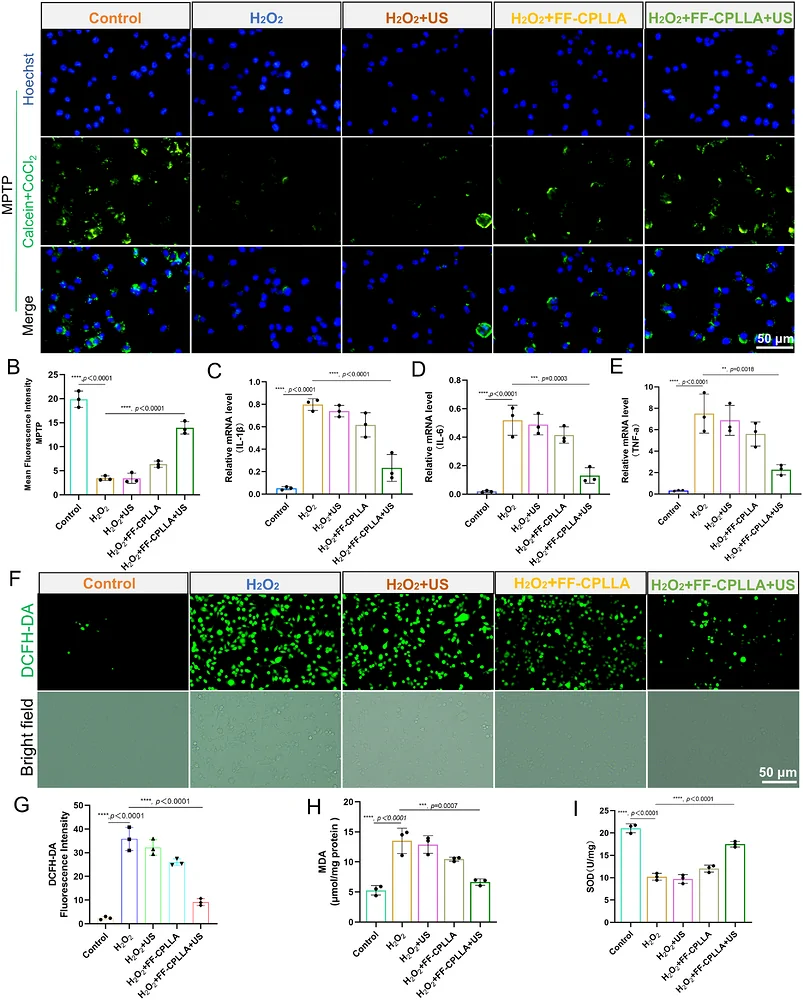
The ultrasound‐responsive FF‐CPLLA piezoelectric fibrous membrane ameliorates MPTP opening, neuroinflammation, and oxidative stress. (A) Representative fluorescence images showing the degree of MPTP opening in different groups, detected by an MPTP assay kit. Scale bar, 50 µm. (B) Quantitative analysis of the mean fluorescence intensity reflecting MPTP opening. Data are presented as mean ± SD; *n* = 3. One‐way ANOVA was used for statistical analysis. (C–E) mRNA expression levels of pro‐inflammatory cytokines (IL‐1β, IL‐6, TNF‐α) measured by RT‐qPCR in different groups. Data are presented as mean ± SD; *n* = 3. One‐way ANOVA was used for statistical analysis. (F) Representative fluorescence images of intracellular ROS detected by the DCFH‐DA probe. Scale bar, 50 µm. (G) Quantitative analysis of the mean fluorescence intensity of DCFH‐DA. Data are presented as mean ± SD; *n* = 3. One‐way ANOVA was used for statistical analysis. (H, I) Levels of MDA and SOD activity measured by commercial assay kits in different groups. Data are presented as mean ± SD; *n* = 3. One‐way ANOVA was used for statistical analysis.
